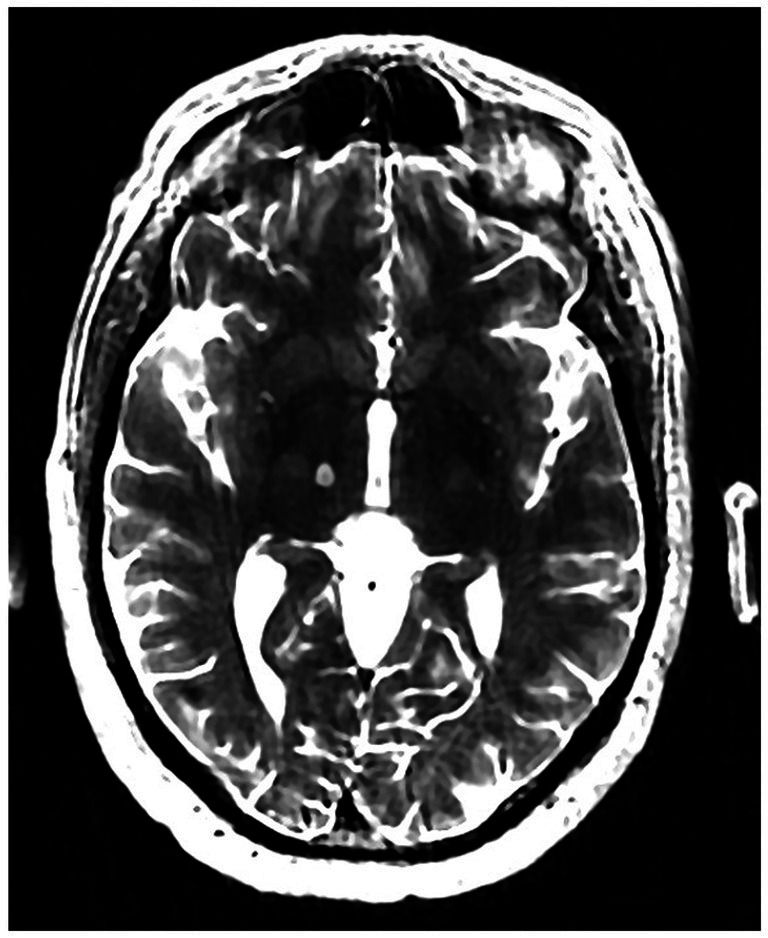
Volumetric T2-weighted image showing right VIM MRgFUS thalamotomy lesion.
